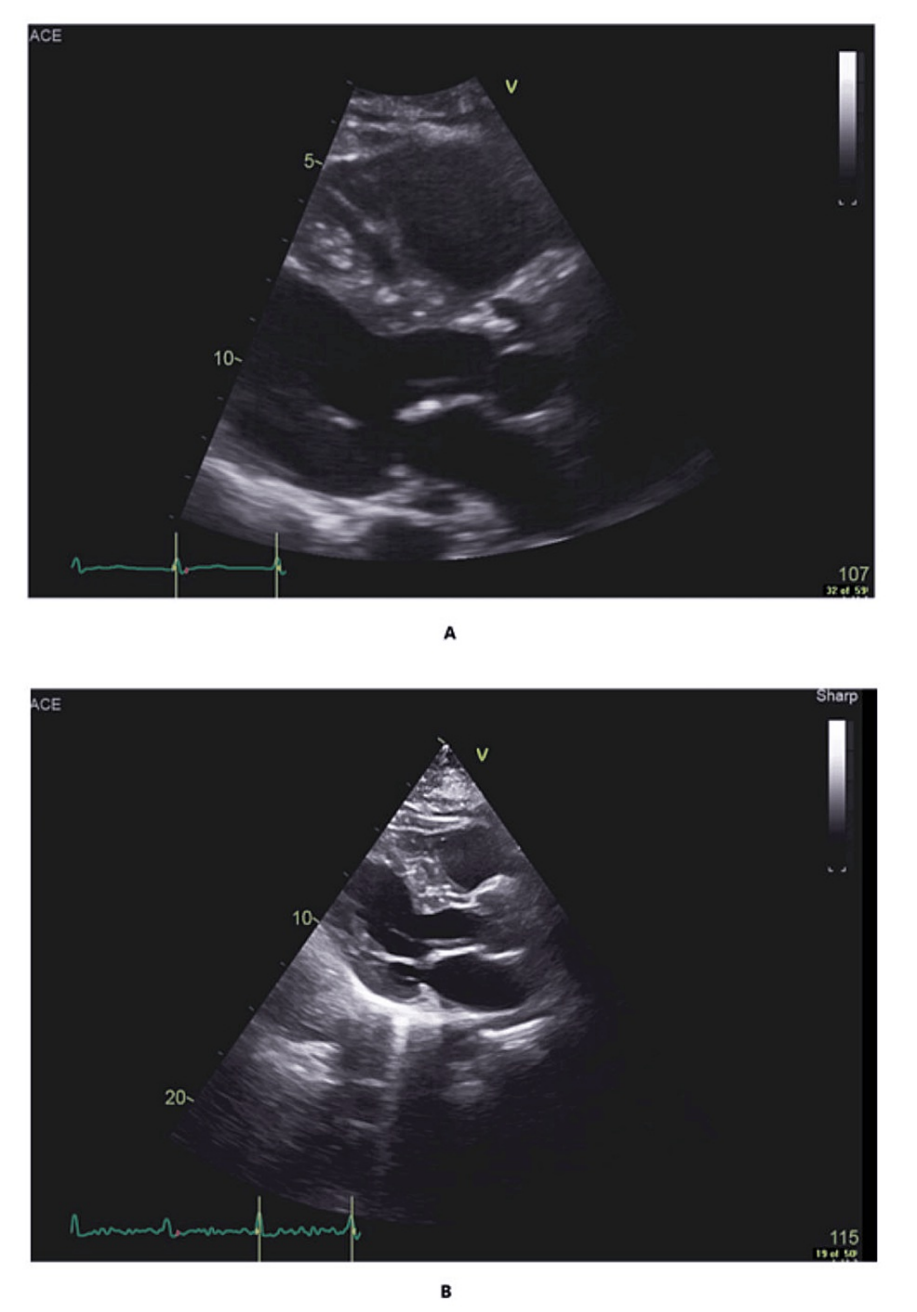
(A) Parasternal view of the transthoracic echocardiogram showing a dilated right ventricle suggestive of right ventricular strain. (B) Previous transthoracic echocardiogram on the chart showing a normal right ventricular pattern (for comparison purposes only).
